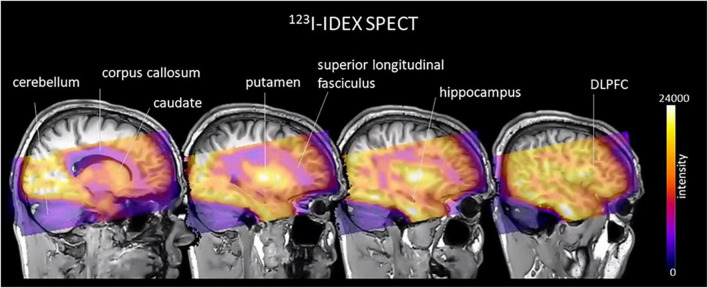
An example of an image, in cool-warm colors, obtained after ^123^I-IDEX SPECT scan registered and overlain onto T1 weighted anatomical MRI scan showing high specific preferential binding to CHRM1 and CHRM4 receptors in the cortex, including the dorsolateral prefrontal cortex (DLPFC), hippocampus, and striatum, with no uptake in the cerebellum, which is devoid of CHRM1 and CHRM4.
